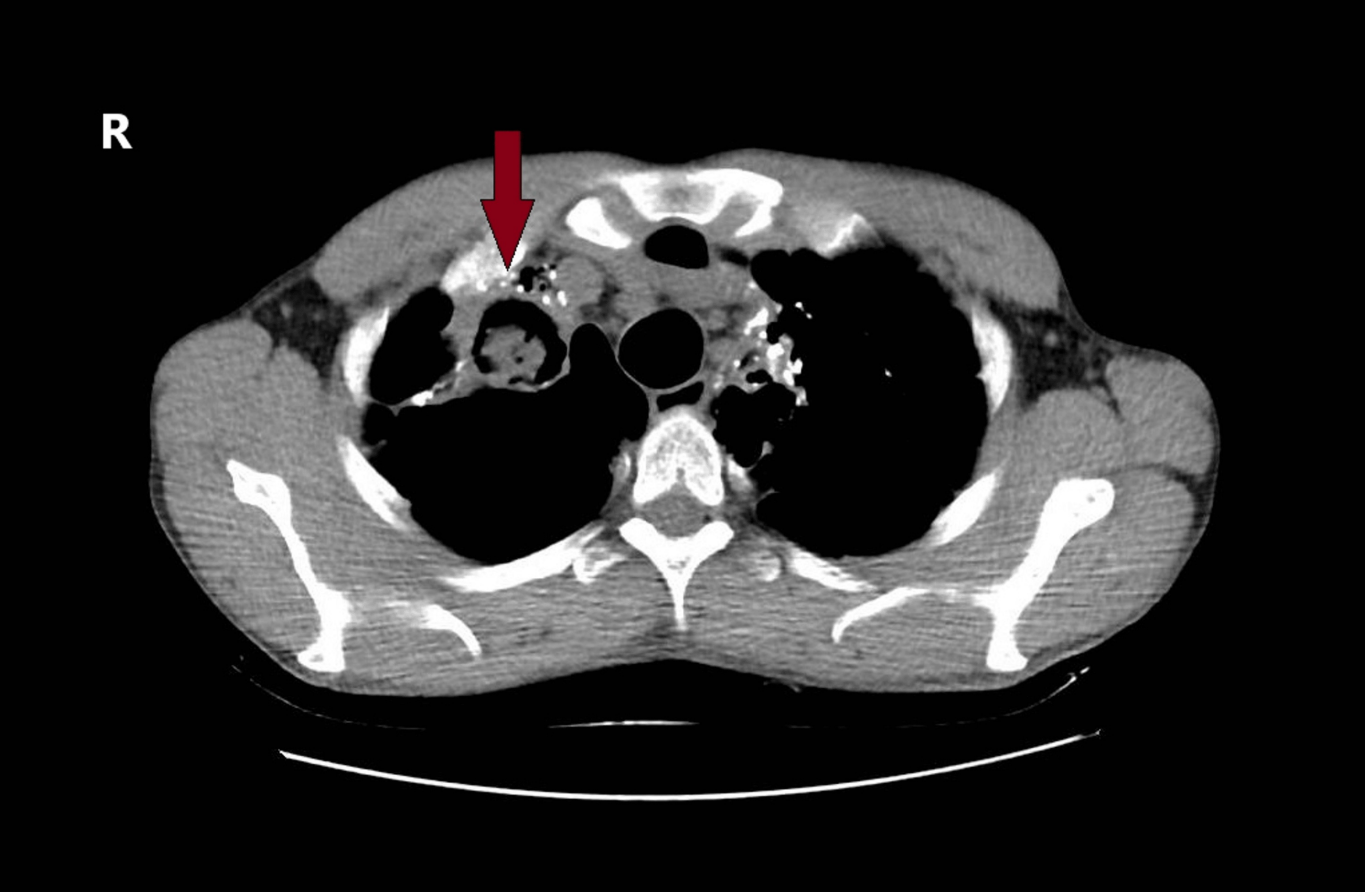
Transverse section of the preoperative thoracic computed tomography scan Right lung cavitary lesion (upper and middle lobes) with typical features of aspergilloma (air crescent sign - a crescent-shaped airspace separating the mass from the cavity wall - red arrow)
